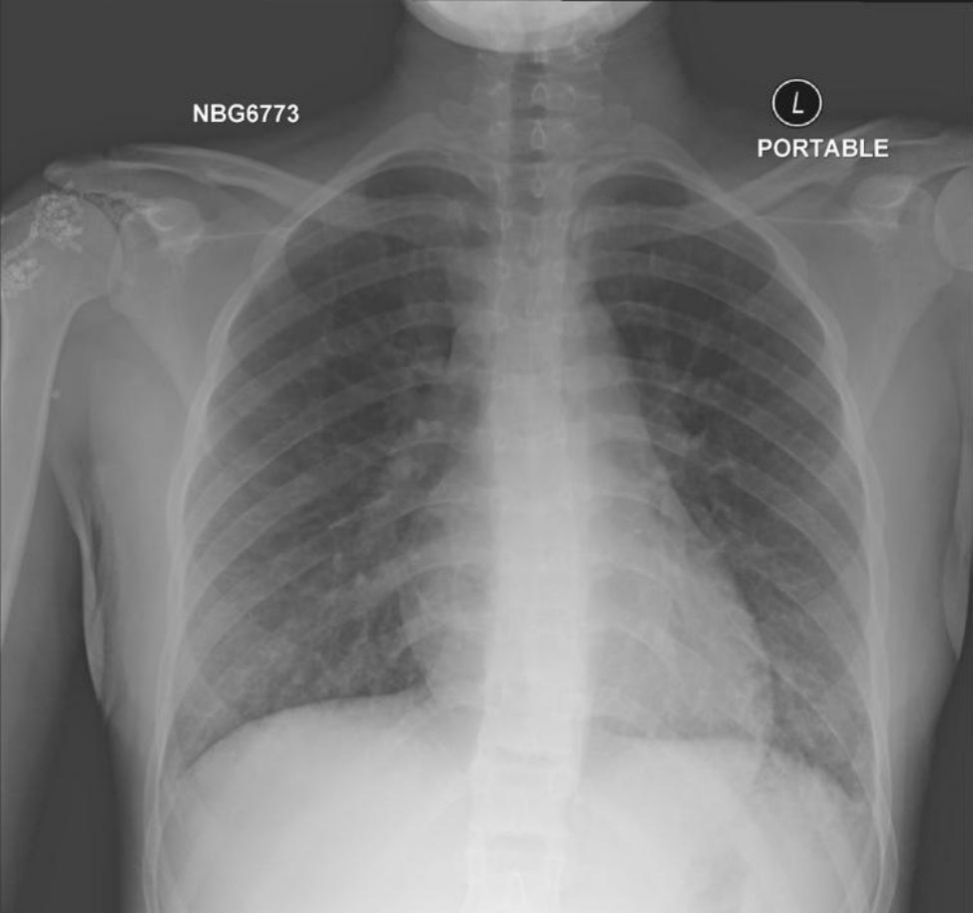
Chest x-ray showing bilateral lower lung zones airspace opacities with subtle left-sided pleural effusion
